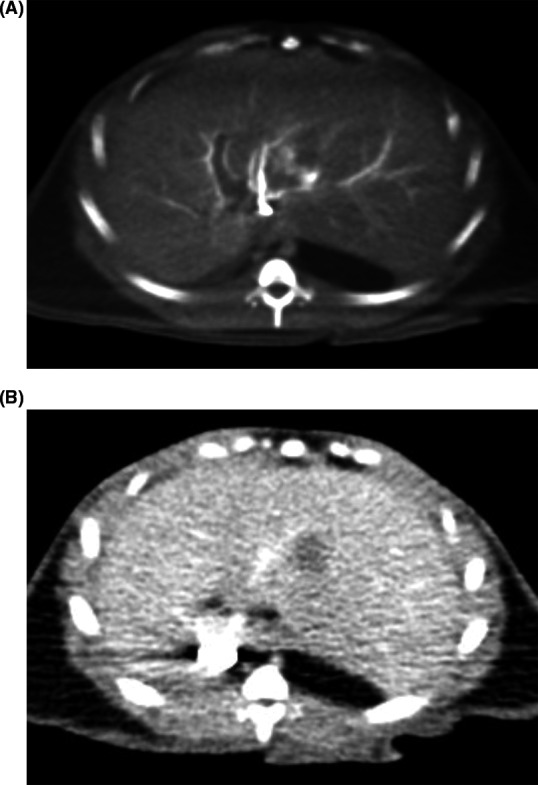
CT findings. A, Post‐contrast CT images demonstrate eccentric enhancement of a left lobe HCC. B, Delayed phase CT images show washout of this mass relative to background liver
